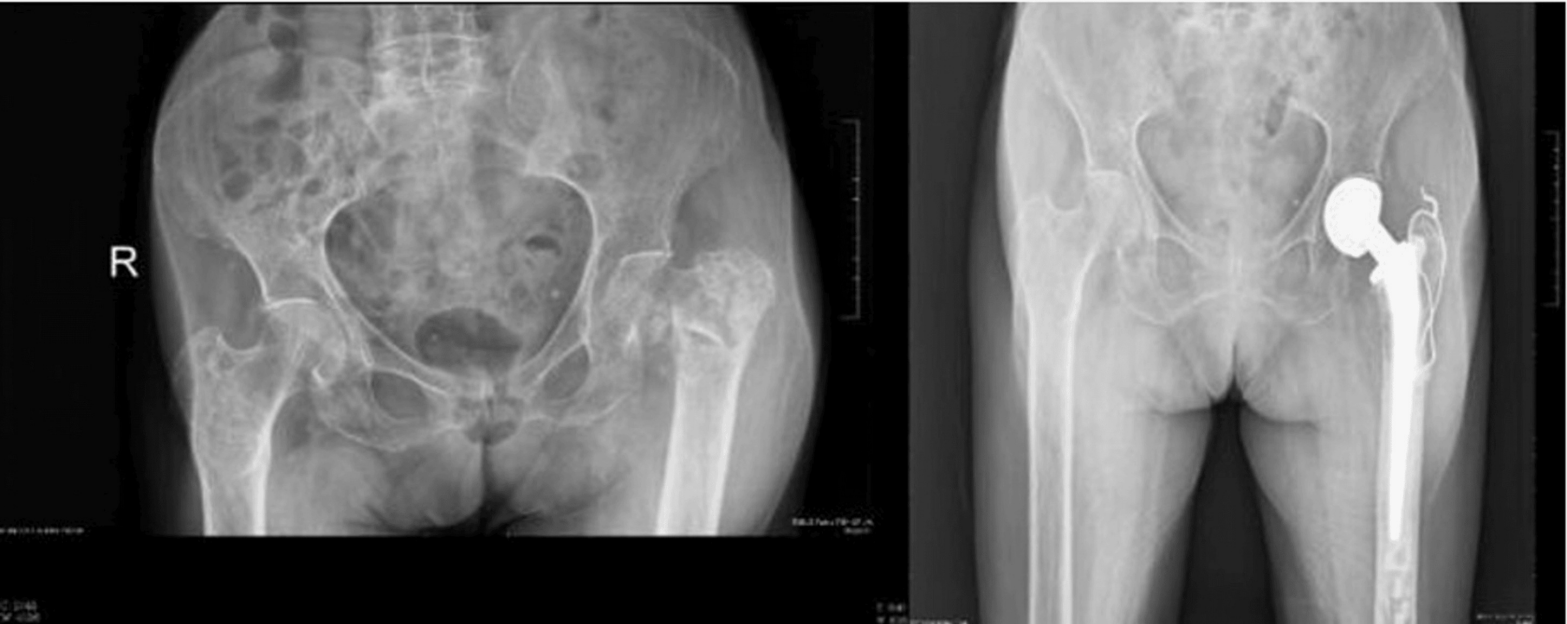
Preoperative and postoperative radiographs Preoperative anteroposterior radiograph of the pelvis showing a right-sided intertrochanteric femur fracture (left image). Postoperative radiograph at the one-year follow-up demonstrating satisfactory alignment, adequate stem positioning, and stable fixation (right image)
